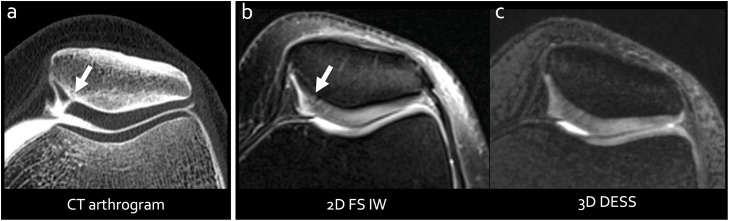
CT arthrogram (spatial resolution: 0.33, reconstructed with a slice thickness of 2 mm) (a), and corresponding 3T MR images (2D fat-saturated intermediate-weighted (2D FS IW)(acquisition parameters: spatial resolution= 0.5 × 0.5 × 3 mm, TR=3000 ms, TE=39 ms (b) and 3D Double Echo Steady State (3D DESS)(acquisition parameters: spatial resolution=0.53, TR=14 ms, TE=5 ms (c) sequences), showing down-to-bone (grade 4) cartilage defect, clearly depicted on CTA (arrow), but which only appears as signal changes on the 2D FS IW sequence and is barely visible on the 3D DESS sequence).
